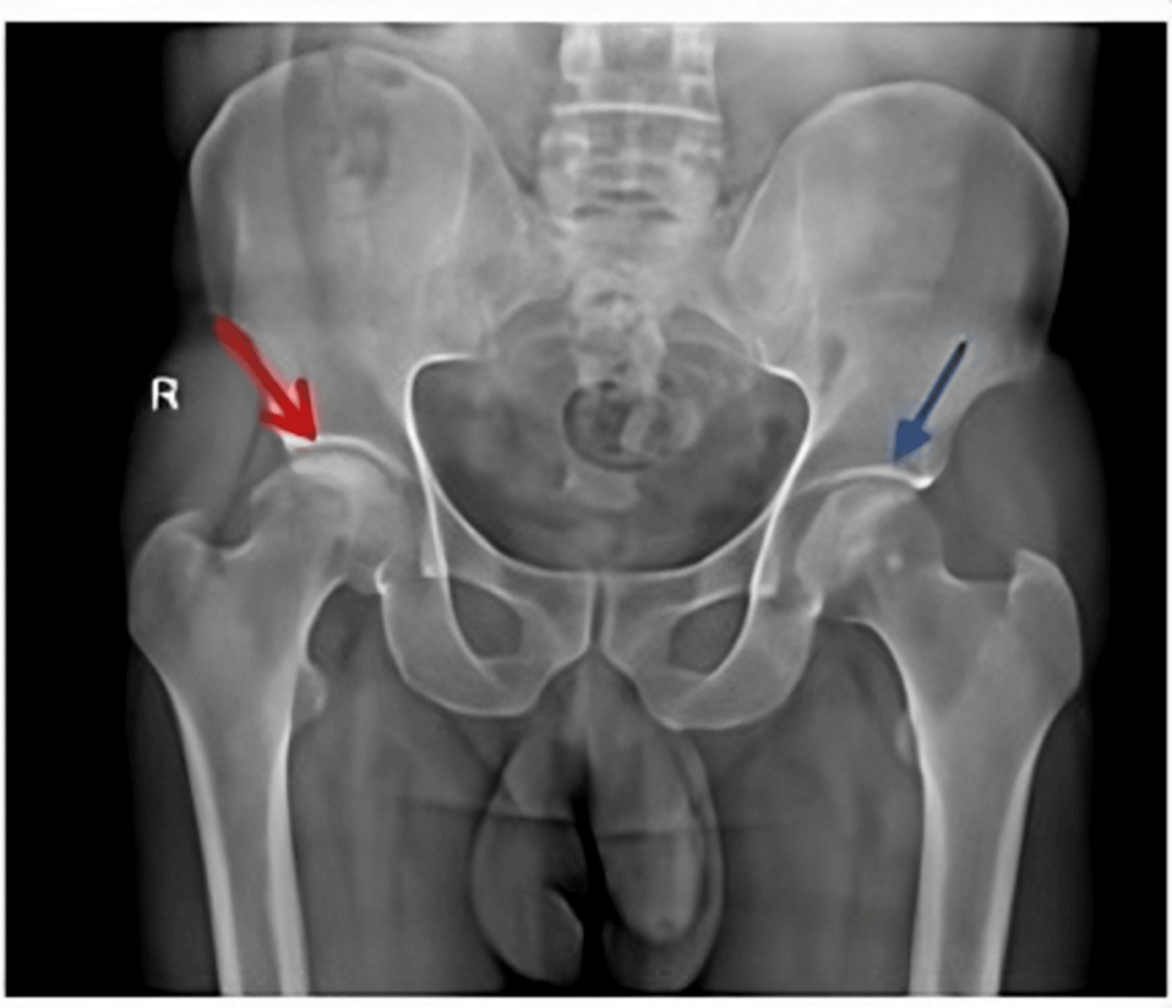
X-ray of the pelvis and both hips of case 1, anteroposterior (AP) view. Findings:Flattening with mild cortical collapse and sclerosis of the right femoral head representing Ficat stage III AVN (red arrow) and minor subarticular sclerosis of the left femoral head representing Ficat stage II AVN (blue arrow). AVN: avascular necrosis
